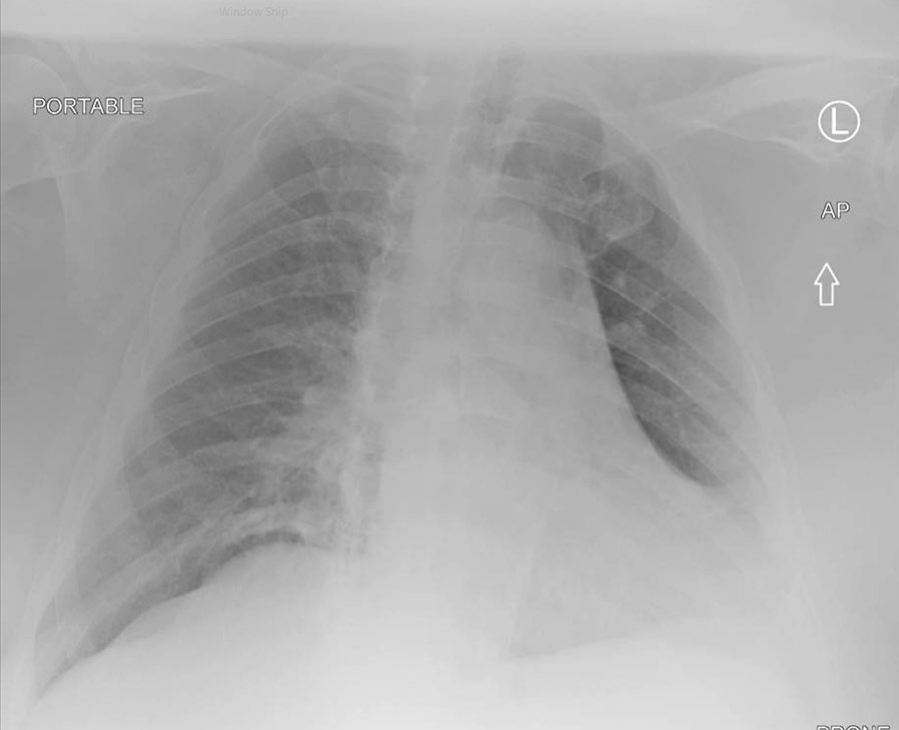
Initial Chest X-Ray in April 2020 with coronavirus disease 2019 infection
